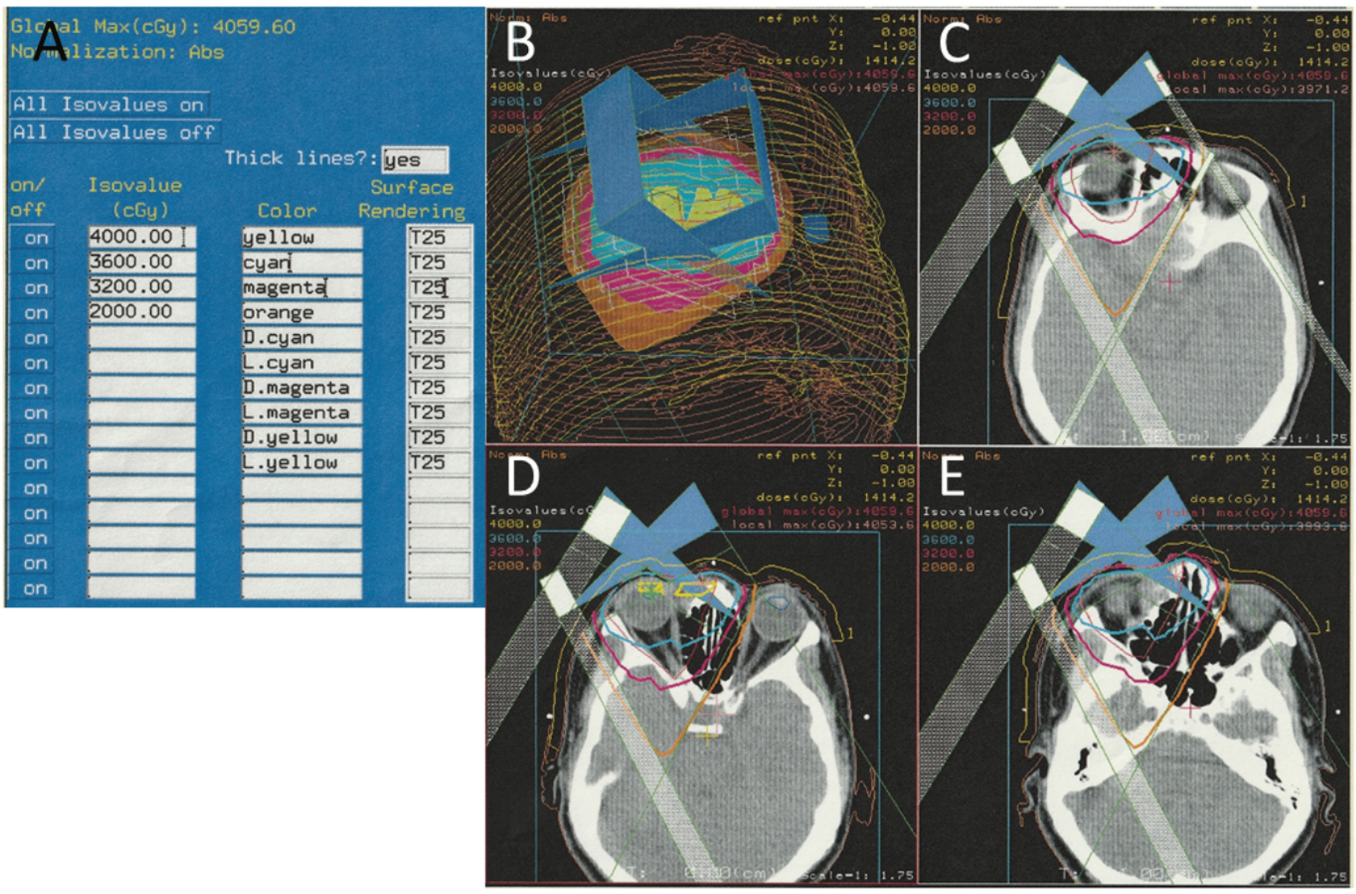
Radiotherapy plan at age 54 years Local radiotherapy plan for primary right lacrimal sac diffuse large B-cell lymphoma at age 54 years. Radiation dose distribution (A) with different color lines (maximum dose at 40 Gy). Front view (B) and different axial sections of computed tomography scans (C, D, E).
